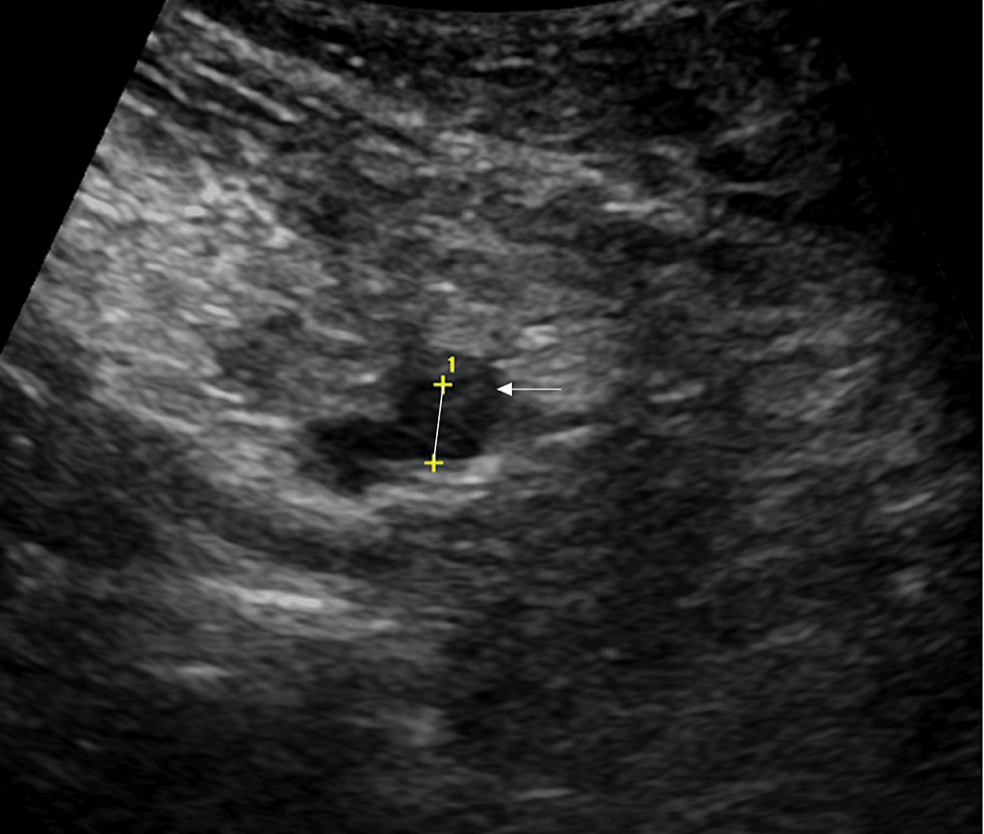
Doppler venous ultrasound with left popliteal deep venous thrombosis (white arrow).
